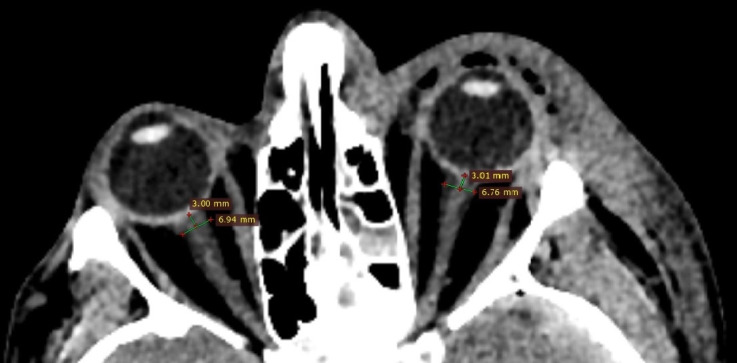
Measurement of optic nerve sheath diameter on axial CT image. The optic nerve sheath diameter is measured 3 mm posterior to the globe, perpendicular to the long axis of the optic nerve, using electronic calipers. The outer-to-outer diameter is recorded. Bilateral measurements are performed, and the mean value is used for analysis.
